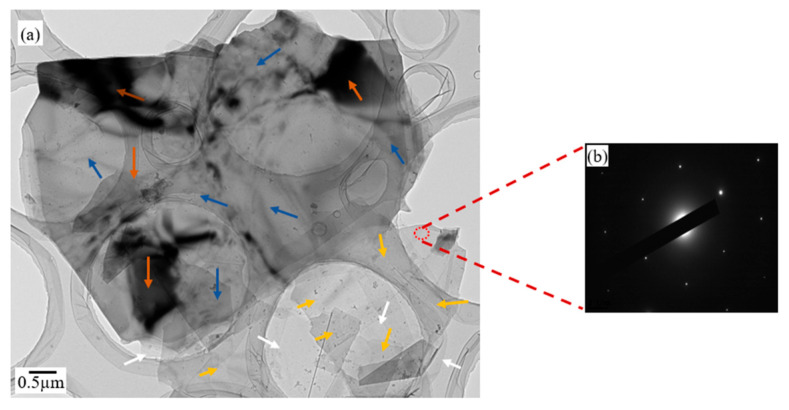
(a) TEM image of graphene platelets after one-hour ultrasonication and (b) electron diffraction pattern of a randomly selected area.
